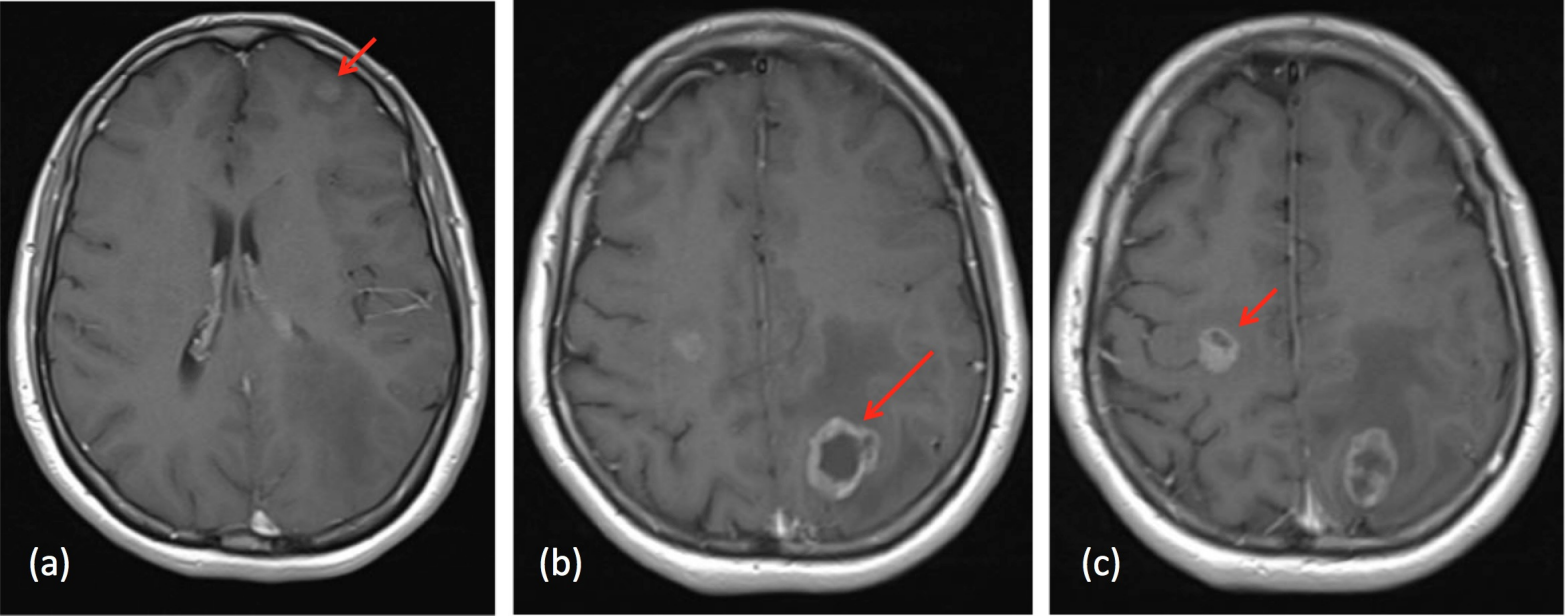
MRI head at diagnosis (November 2014) (a) 11 x 9.9 x 9.9 mm left frontal BM; (b) 24.3 x 25.3 x 22.2 mm left parietal BM; (c) 14.3 x 11.7 x 17.3 mm right frontal BM MRI: magnetic resonance imaging; BM: brain metastases
